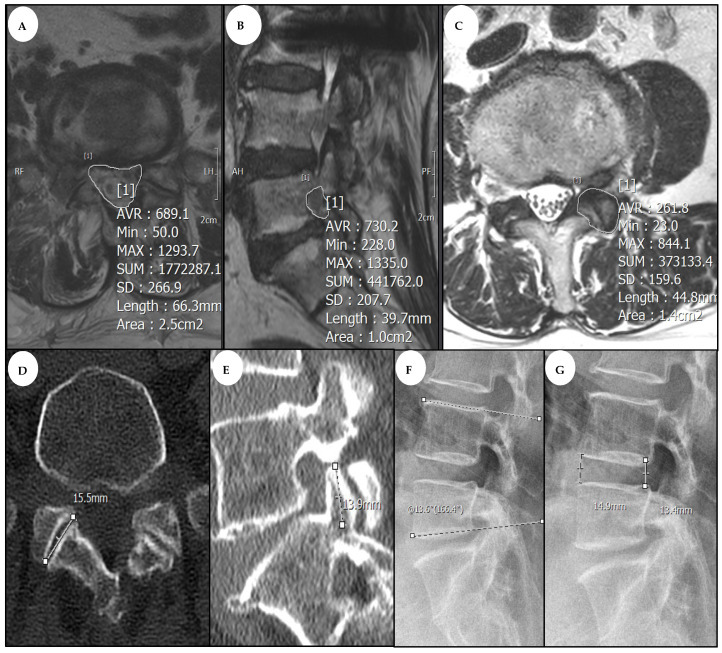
Measurement of radiologic parameters. (A) Measurement of CSA-SC in MRI T2 axial view. (B) Measurement of CSA-IVF in MRI T2 foraminal sagittal view. (C) Measurement of CSA-FJ in MRI T2 axial view. (D) Measurement of facet length in CT axial view. (E) Measurement of SAP length in CT foraminal sagittal view. (F) Measurement of segmental lordosis in lumbar spine lateral plain X-ray. (G) Measurement of disc height in lumbar spine lateral plain X-ray.
